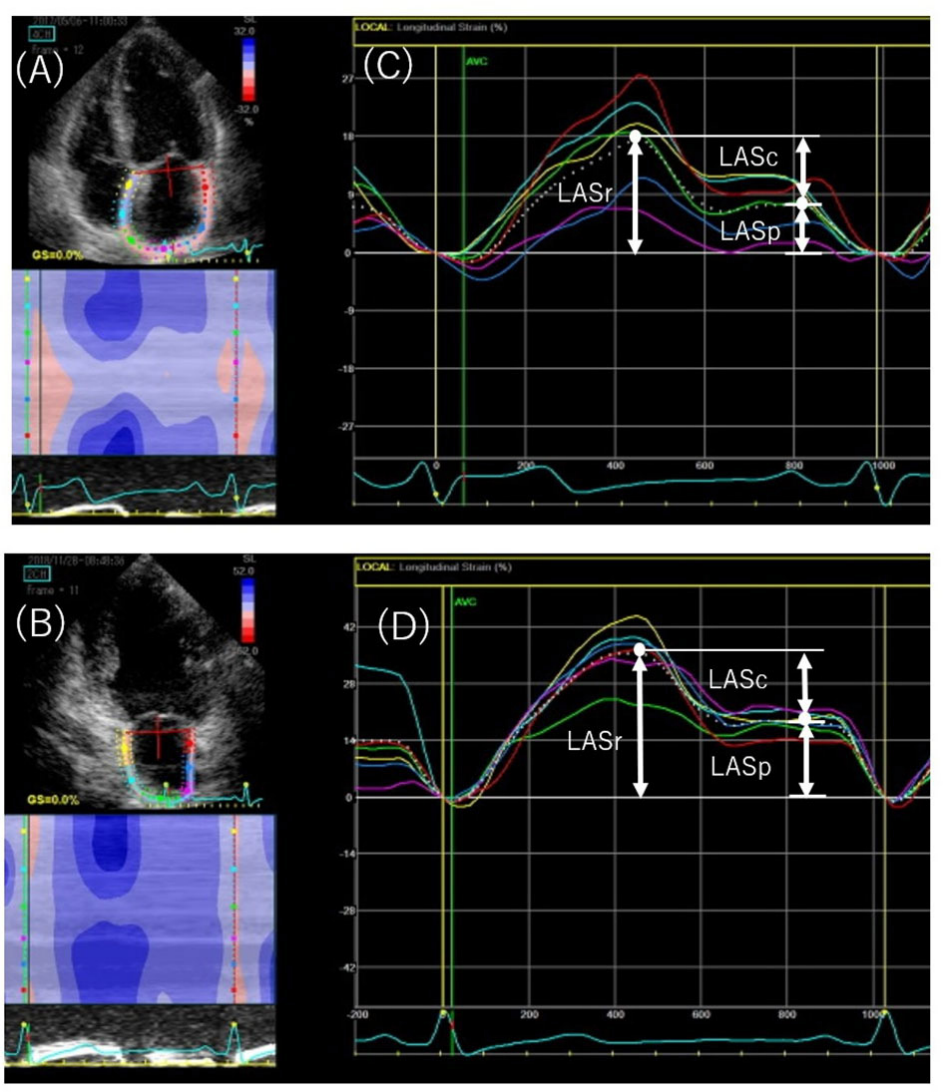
Speckled-tracking echocardiography to assess left atrial (LA) phasic strain. Endocardial region of interest in the apical 4-chamber (A) and 2-chamber (B) views. The peak positive longitudinal strain (LASr) corresponds to LA reservoir function, and the strain during early and late diastole (LASc and LASp, respectively) corresponds to conduit and pump function in the apical 4-chamber (C) and 2-chamber (D) views. The white dotted curved line indicates the average of LA strain. LASr, peak left atrial strain during ventricular systole; LASc, peak left atrial strain during early diastole; LASp, peak left atrial strain during atrial systole.
